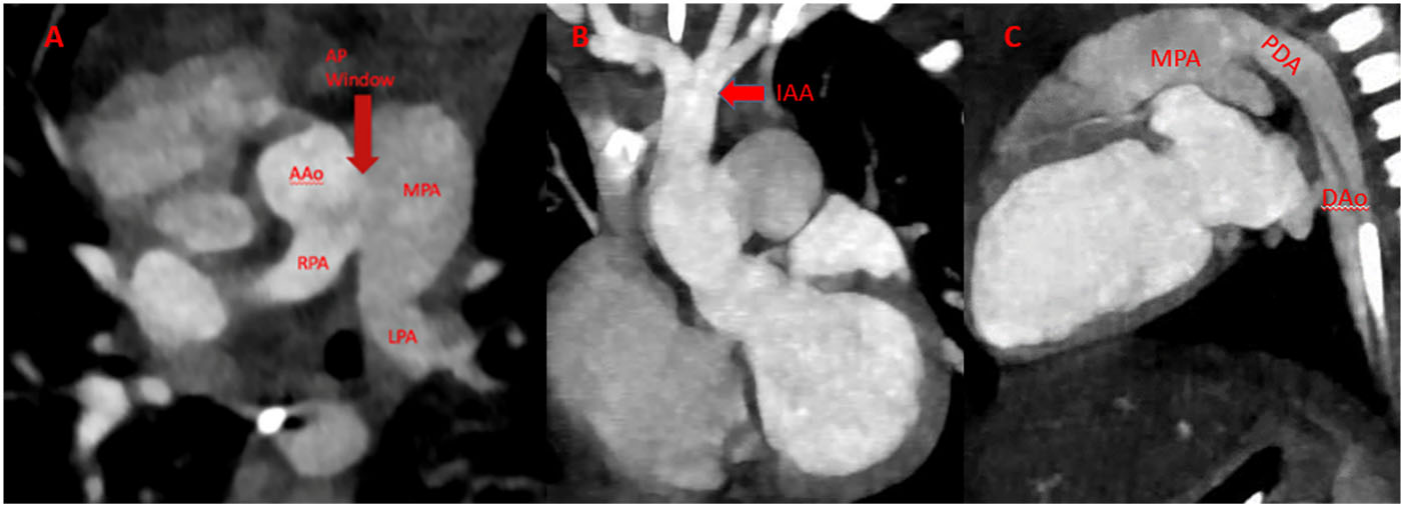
Computed tomographic images demonstrating: (A) a type III aortopulmonary
